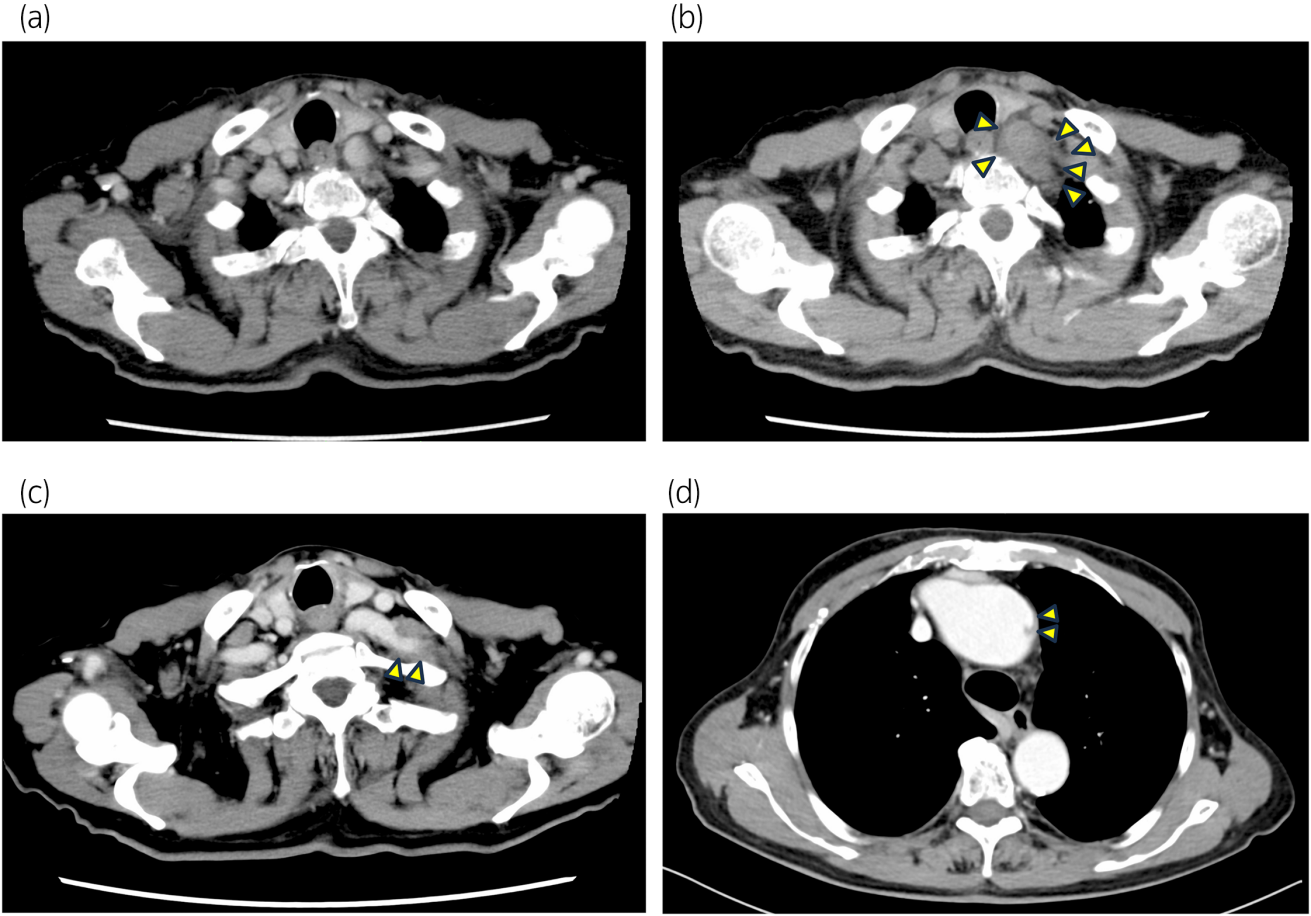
Computed tomography images. (a) Image obtained before the first administration of filgrastim. (b) Aortic arch wall thickening is observed after filgrastim administration. (c) Left subclavian artery wall thickening is reported following pegfilgrastim administration. (d) A small aortic dissection of the aortic arch is noted 6 months after the first chemotherapy cycle.
